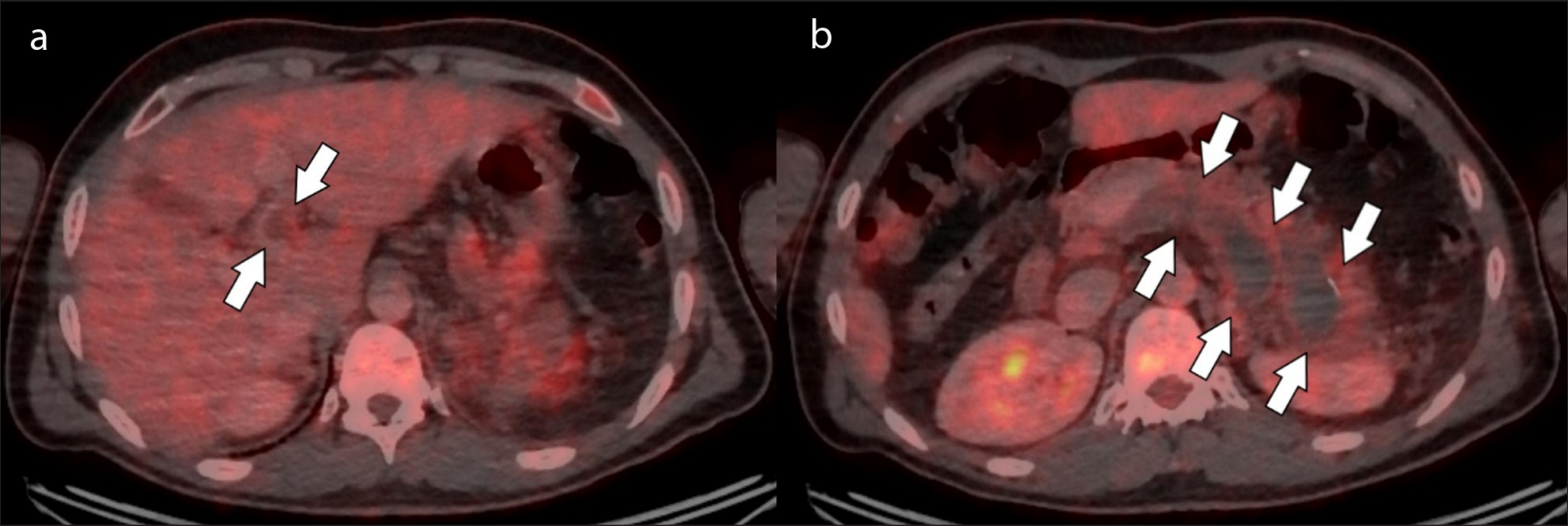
Axial FDG-PET/CT slices at the level of the splenic vein (Figure 2a) and portal vein (Figure 2b) showing absence of metabolism within the thrombus and some hypermetabolic infiltration of surrounding fat (arrows).
